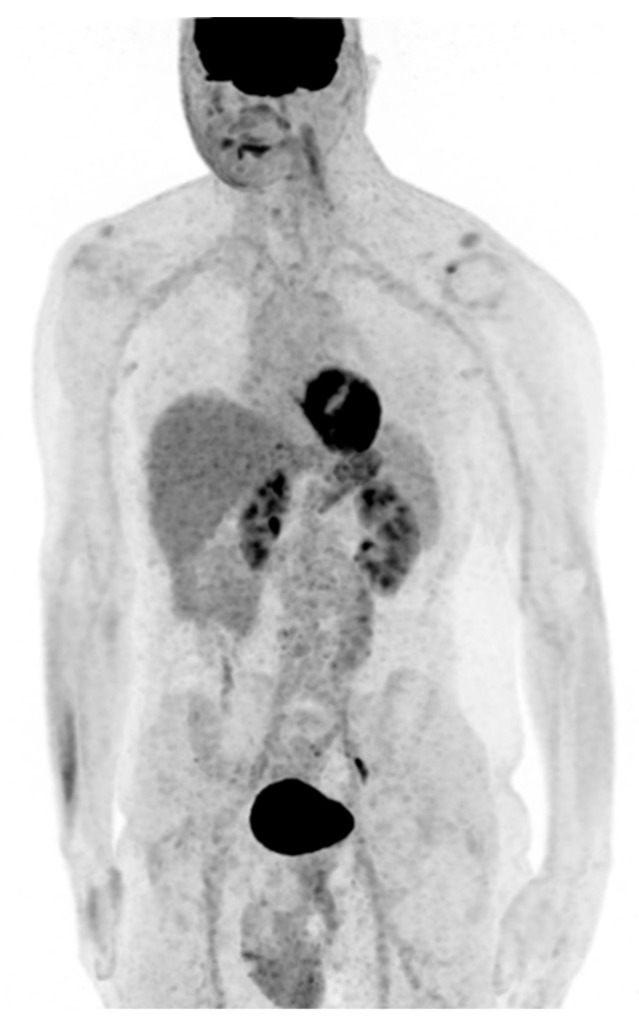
Post-surgical PET/CT with ^18^F-FDG does not show pathological uptake.
